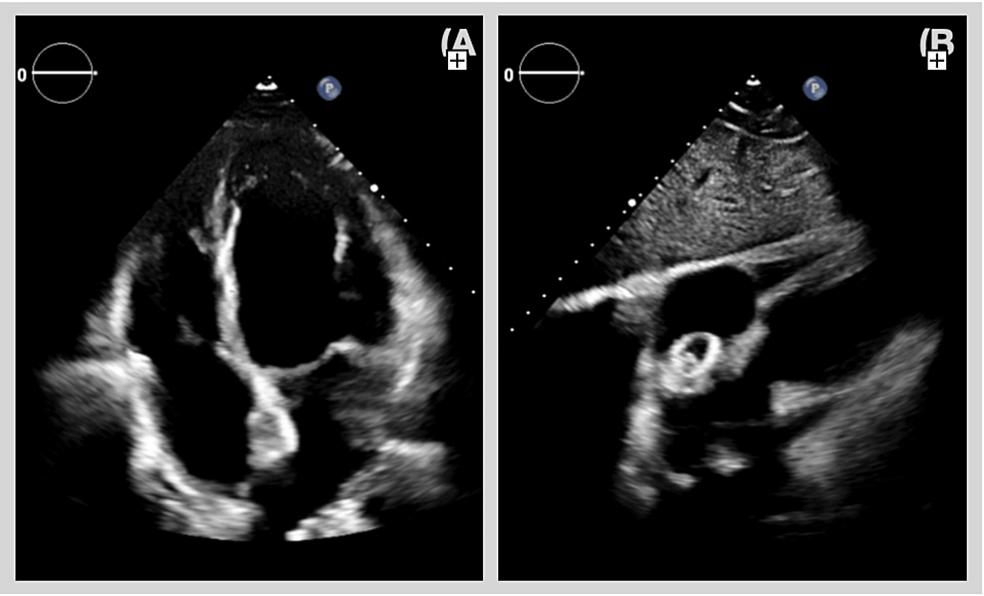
Transthoracic echocardiography: (A) apical four-chamber view and (B) subcostal view, showing an atrial septal occluder device.
